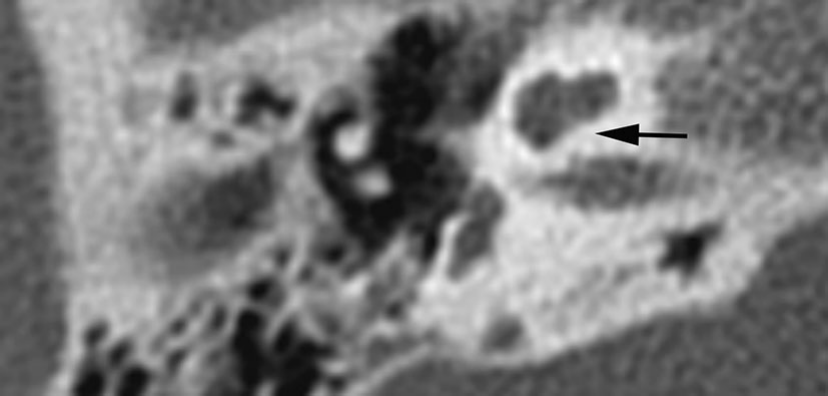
Cochlear aperture—axial CT image shows a lacking cochlear aperture
